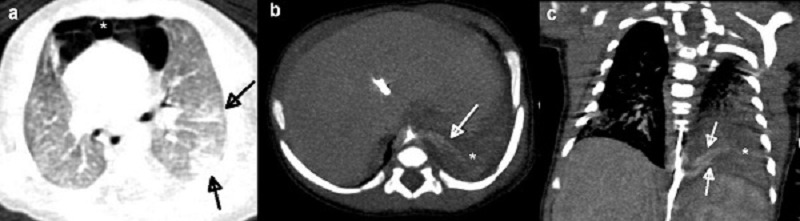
Figure 2: Contrast enhanced chest CT images of the newborn. (a) Axial plane CT in lung window reveals multiloculated, multiseptated, anterior mediastinal air collection between the pericardial sac and the thymus compatible with pneumomediastinum (asterix). Particularly, there are some ground glass opacities and reticulonodular opacities indicating an infectious process in the inferior lobe of the left lung (black arrows). Axial plane reconstructed Chest CT in mediastinal window (b), demonstrates the anomalous mass like tissue which is the pulmonary sequestration located in the left lower lobe (asterix). It has a systemic arterial supply which is a branch of the aorta (white arrow). On coronal view (c), note the pulmonary sequestration (asterix) and other vascular structure parallel to the artery (white arrow) which is a draining vein to the azygos vein confirmed by Doppler sonography.
